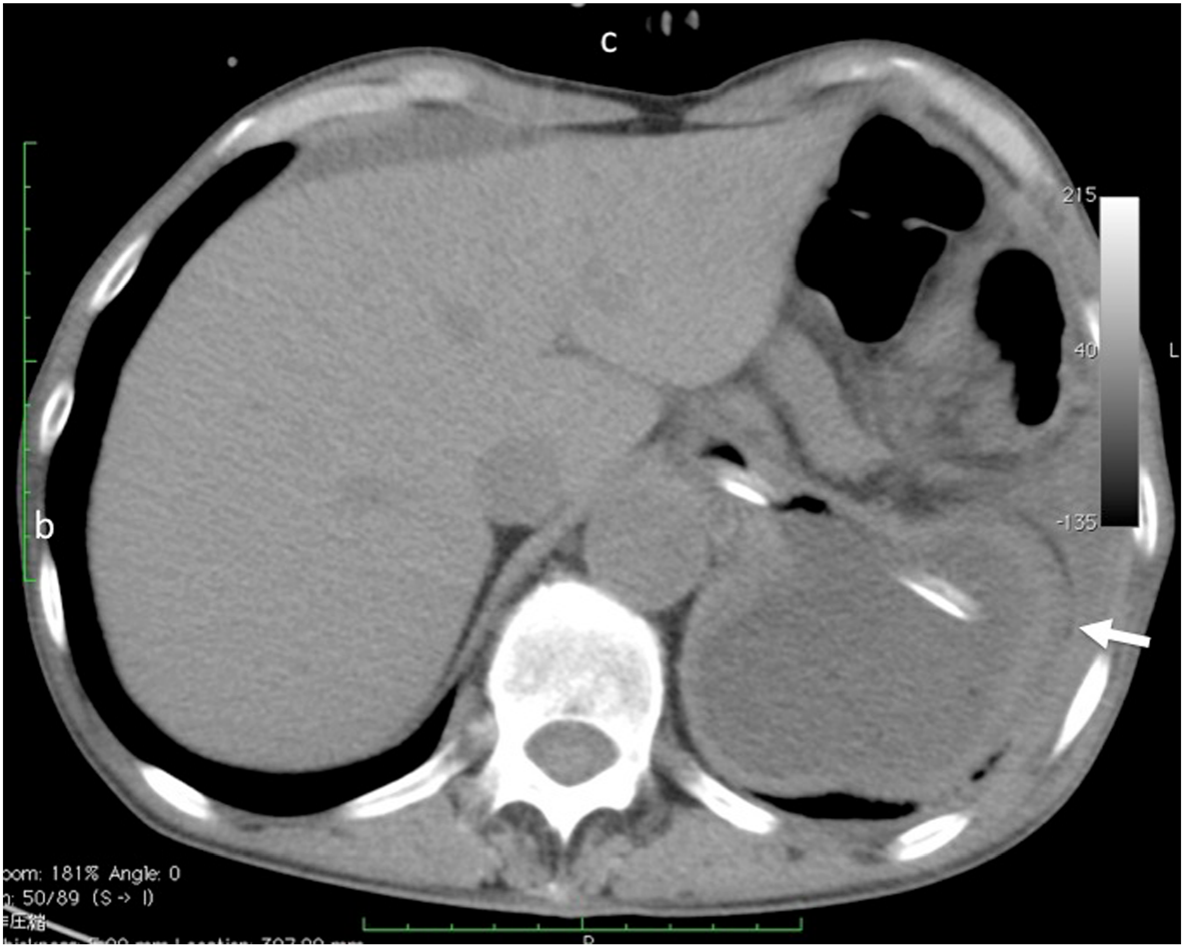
Chest computed tomography showing a large fluid-filled structure in the retrocardiac space. Mild left pleural effusion and atelectasis are indicated.
